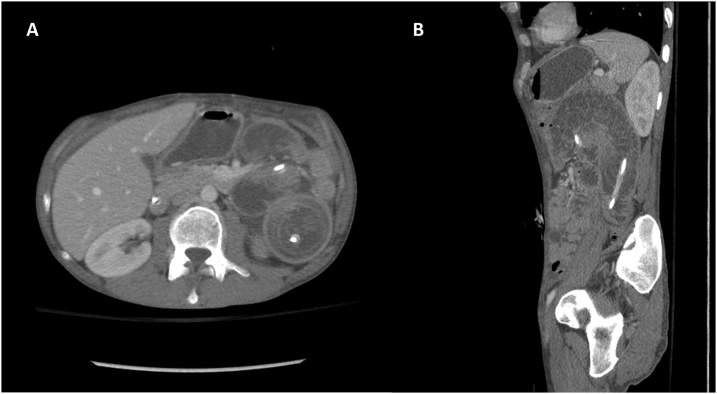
(A) Abdominal CT showed Jejunojejunal intussusception with distension of stomach and duodenum. (B) Gastrojejunal tube was passed through intussusception.
